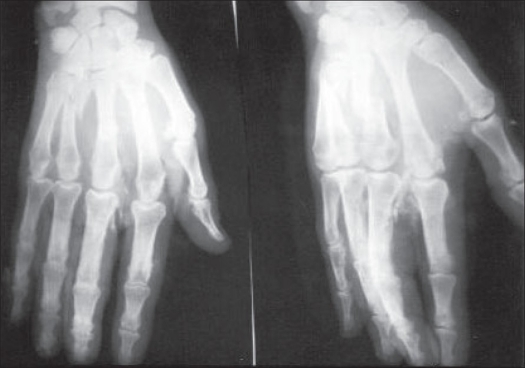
Preoperative radiographs showing open fractures of III^rd^ and IV^th^ metacarpal
